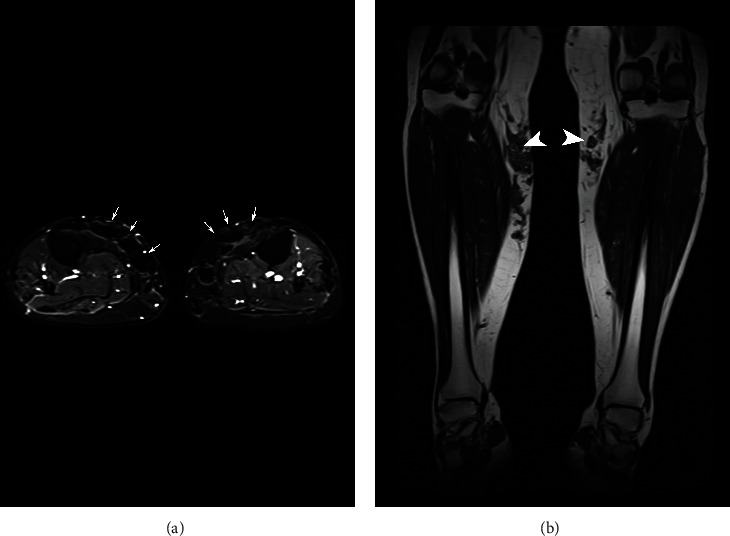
Magnetic resonance imaging using STIR (short tau inversion recovery, also known as short-TI inversion recovery) sequence. STIR is a fat suppression technique with an inversion time TI = ln(2) · T1fat, where the fat signal is zero; this equates to approximately 140 ms at 1.5 T. STIR is used to distinguish two tissue components when their T1 values are different. It allows homogeneous and global fat suppression and can be used with low-field-strength magnets. However, this technique is not specific for fat; thus, the signal intensity of tissue with a long T1 and tissue with a short T1 may cause ambiguity. In this figure, STIR shows in two planes the infiltration by the modeling agent to the legs, an unknown substance, 800 ml. (a) Axial plane: the presence of modeling material with a hypointense globular pattern that affects the anteromedial surfaces of the subcutaneous cell tissue of both legs is observed (white arrows). (b) Coronal T1 sequence: the signal of the modeling material is isointense to the muscle (white arrowheads).
